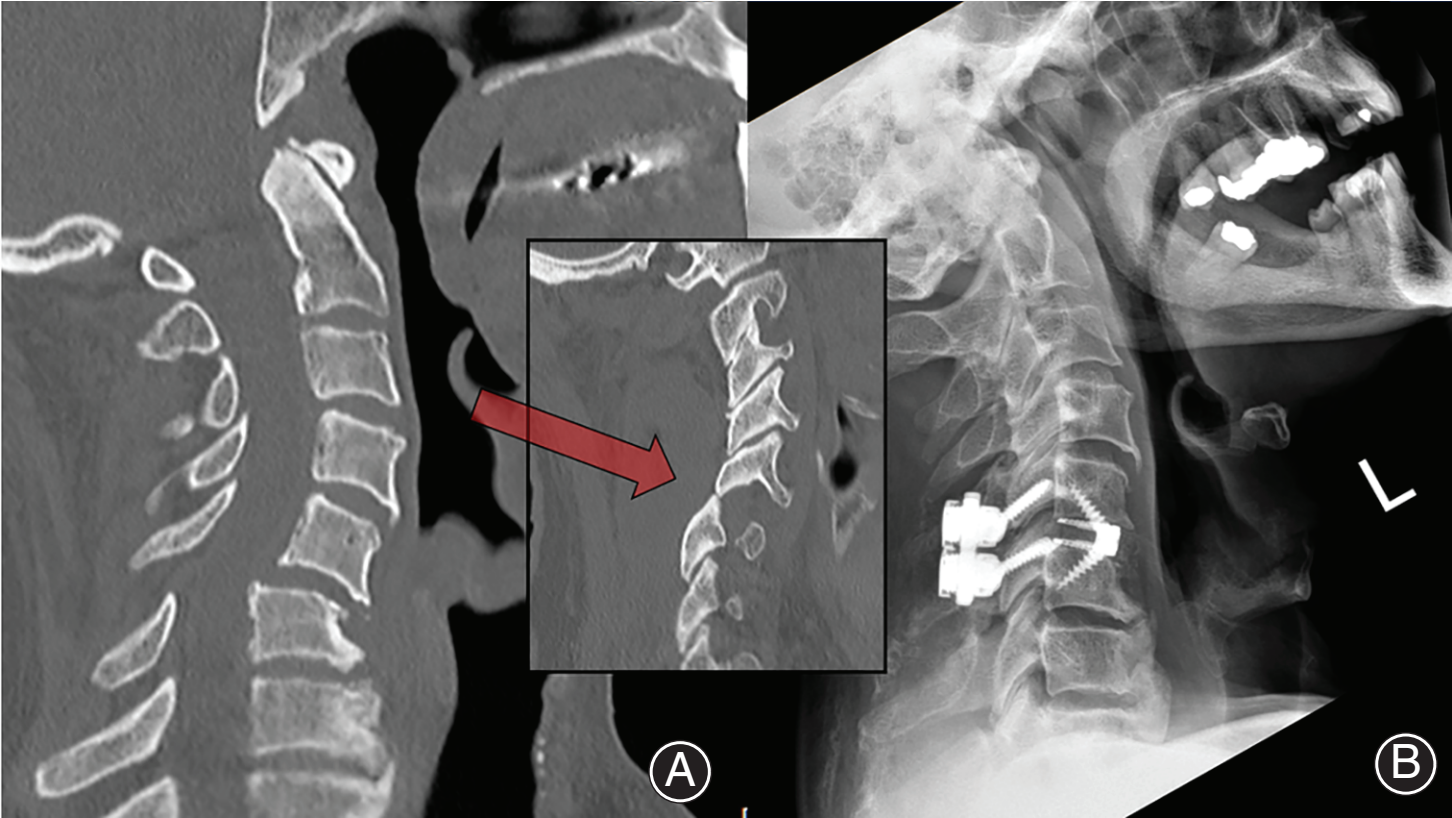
(A) Pre‐operative CT scan showing trauma with (inset) Bifacetal dislocation at C_5‐6_. (B) Anterior interbody fixation, with posterior lateral mass fixation (Neon 2 Posterior Cervical, Ulrich, Germany).
